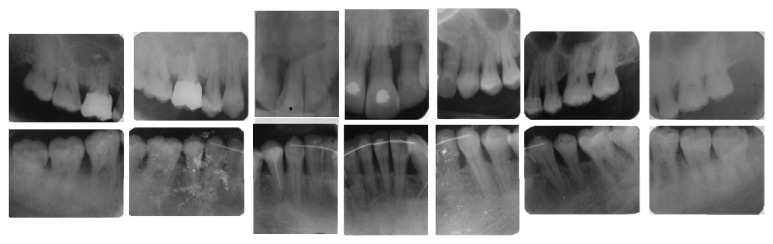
Full mouth radiographs demonstrating generalized severe horizontal and angular bone loss.
